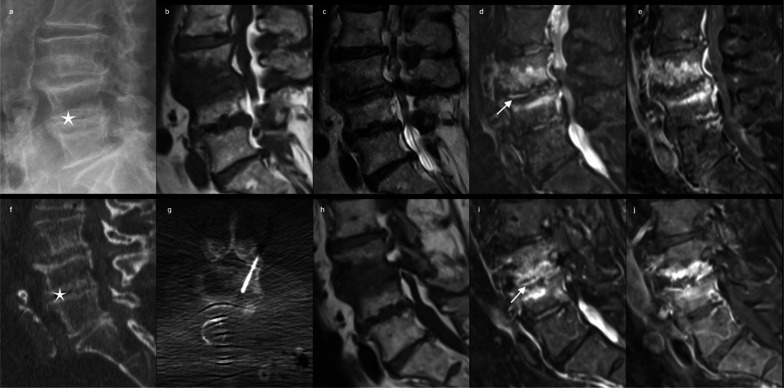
68-year-old male with increasing back pain referred for pathologic confirmation and treatment of spondylodiscitis. Sagittal XR (a), Sagittal T1 (b), T2 (c), STIR (d) and T1 Fatsat after gadolinium R1 point 5 (e) confirm edema-type enhancing marrow signal abnormalities as well as disc hyperintensity (arrow), and narrowed L3–L4 space without disc enhancement. Following sagittal CT (f) R1 point 6 confirms the absence of endplates destruction and that the hypersignal on the underlying disc (L4–L5) corresponds to the vacuum phenomenon (star), biopsy performed under CT guidance (g) was negative. At 3 months, MRI control on sagittal T1 (h), STIR (i) and after contrast (j) shows stability of disco-vertebral features (arrow)
